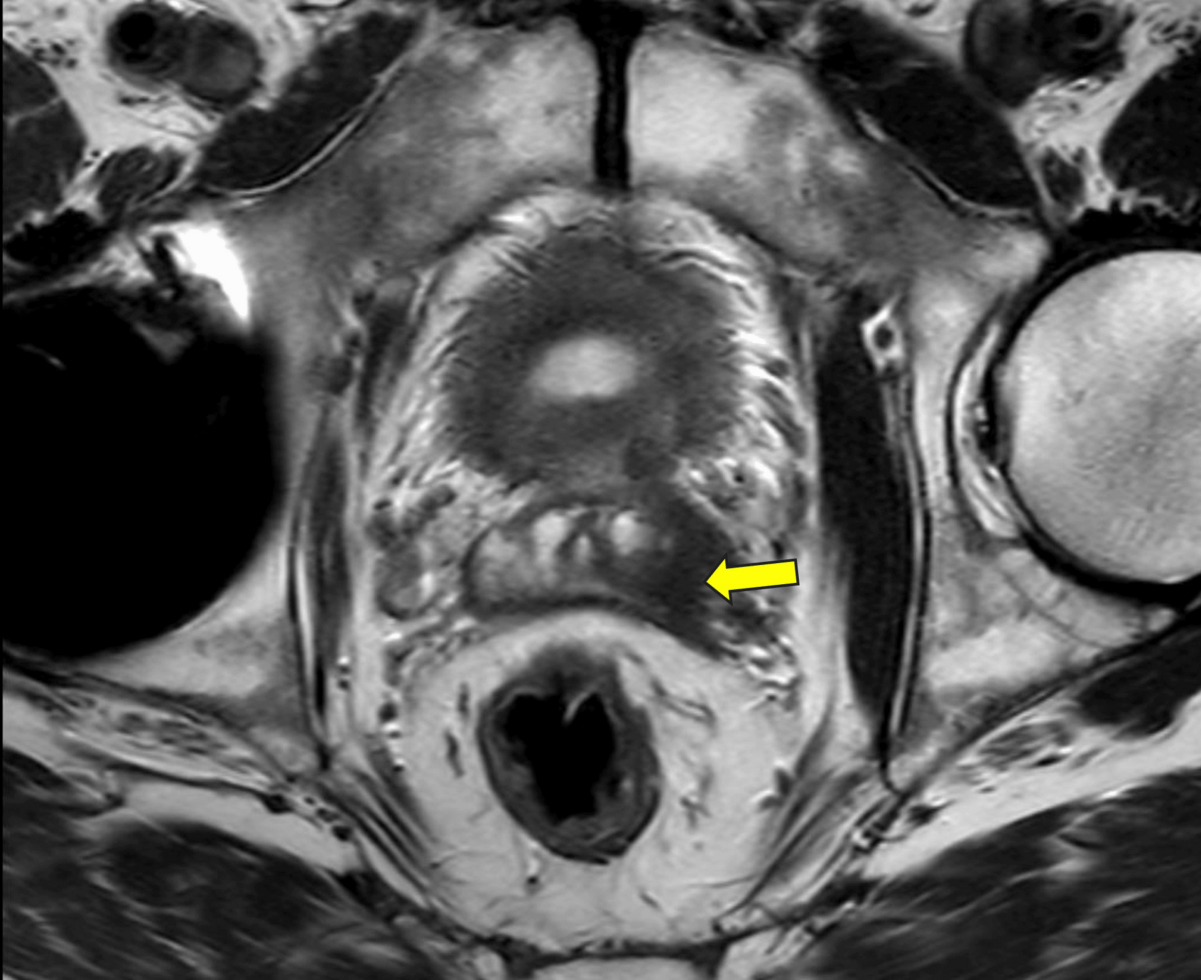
Axial T2 turbo spin echo (TSE) MRI image with the yellow arrow pointing to the hypo-intense area involving the left seminal vesicle, suspicious for disease.
